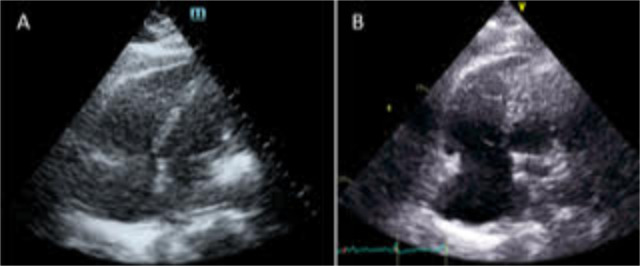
A) E-POCUS, B) Comprehensive transthoracic echocardiogram (CTE) A case of a 75-year-old woman presenting to ER with acute shortness of breath and desaturation. E-POCUS and CTE revealed significant RV dilatation with severe systolic dysfunction.
